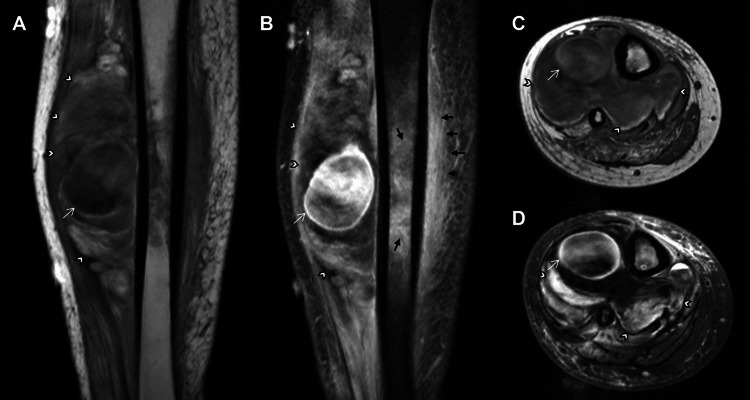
Right Lower Extremity MRI showing pseudoaneurysm (A) Coronal pre-contrast, (B) Coronal post-contrast, (C) Axial pre-contrast, and (D) Axial post-contrast T1W MRI showing 3.9 cm pseudoaneurysms (white arrows) in the anterolateral compartment of the right lower extremity calf. There is a sizeable hematoma (white arrowheads) with surrounding soft-tissue enhancement and intramedullary enhancement (black arrows) in the subjacent tibial diaphysis. Note: Pulsation artifact is observed within the pseudoaneurysm.
